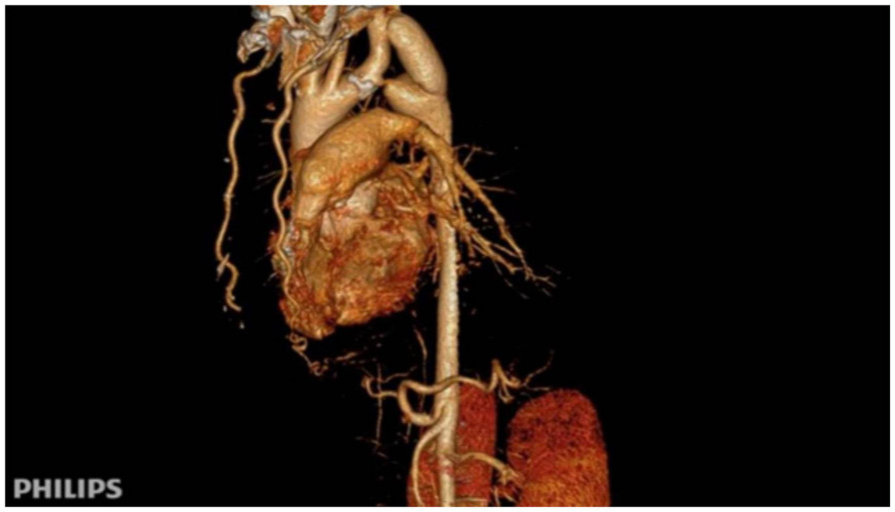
Immediate postoperative CTA following subclavian-to-descending aorta bypass. Patency of the synthetic vascular graft (Dacron) from the left subclavian artery to the descending aorta is demonstrated, with normalization of the aortic lumen distal to the coarctation.
